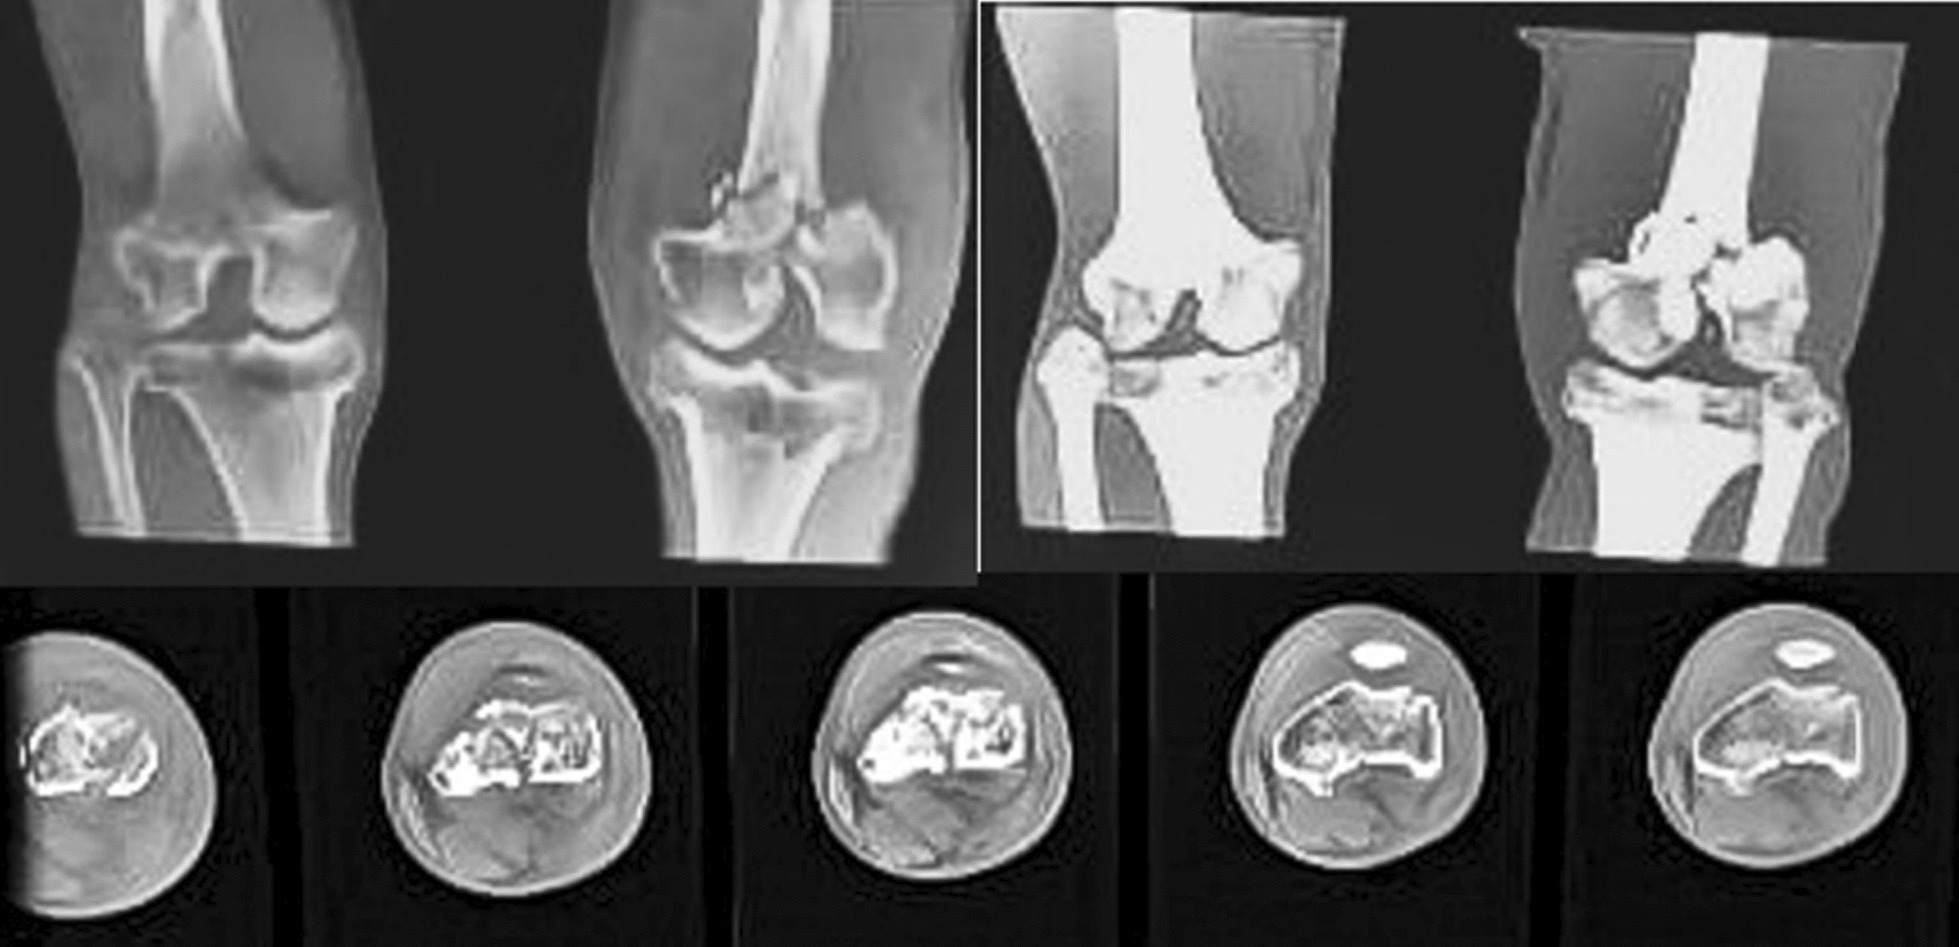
Computed tomography of the left knee showing the fractured distal femoral condyles
